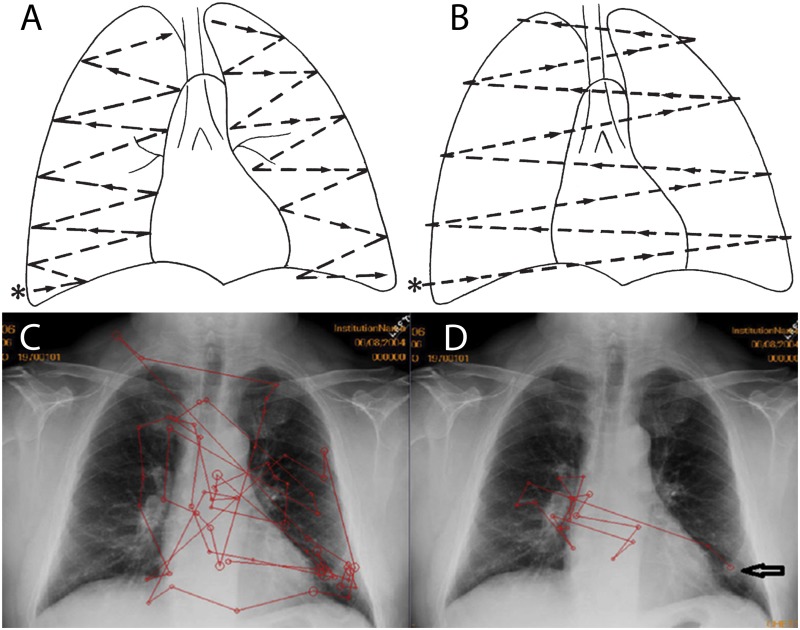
Reprinted from Goodman and Felson (2007) with permission. (A,B) Proposed lung search pattern. (A) This commonly taught search pattern for examination of the lungs during chest radiograph (CXR) interpretation involves starting at the right base (^∗^) (the costophrenic angle) and examining the right and then left lungs. (B) A second look is then performed in order to compare the right and left lungs as bilateral symmetry is assumed to be useful in recognizing abnormalities (Carmody et al., 1984). Reprinted from Waite et al. (2017a) with permission. Typical scanpaths of a novice (C) and an expert (D) radiologist, both searching a CXR which has a nodule at the left base (arrow in D). This free search pattern (D) is typically employed by experts and differs from the formal radiologic training given in residency. Instead, it indicates the flexible use of search strategies as a function of immediate visual information. The expert radiologist (D) has more efficient scanpaths (red lines) than the novice (C), with fewer fixations (circles), less coverage of the image, fewer saccades, and faster arrival at the abnormality.
